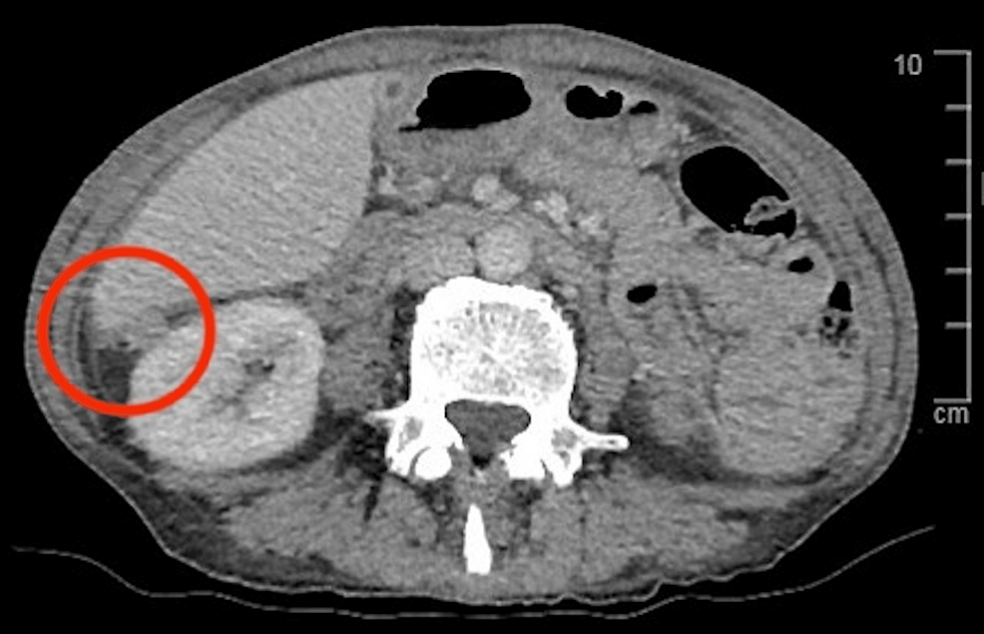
CT demonstrating multi-focal enhancing soft tissue densities in the peritoneum Soft tissue density nodule is present close to the liver, measuring 15 mm.
